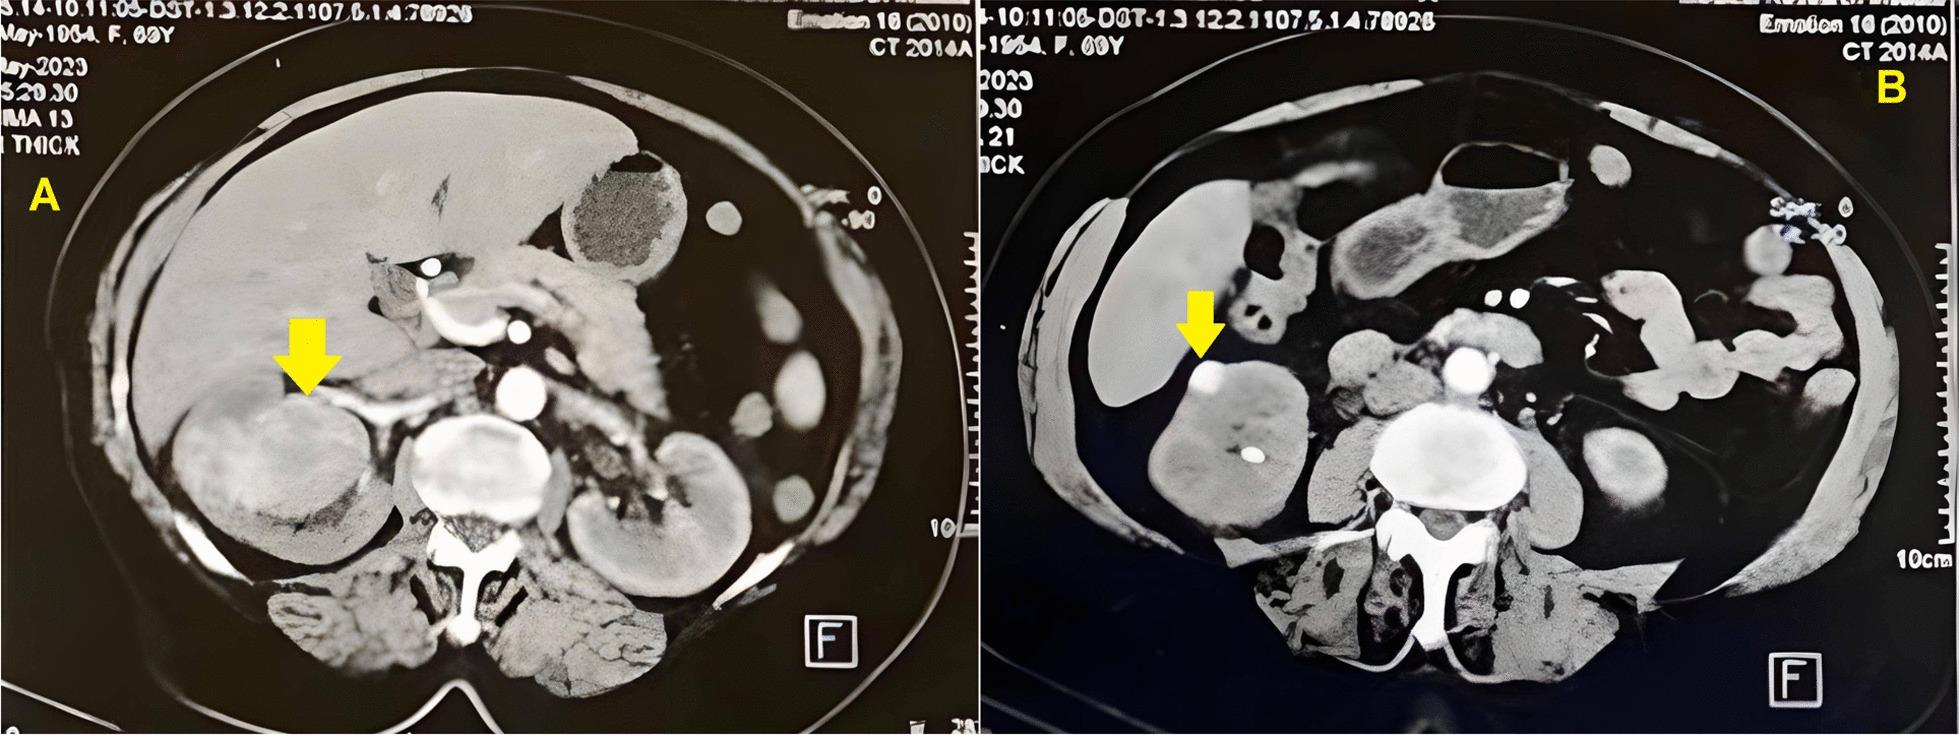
Multi-slice computed tomography displaying two right renal masses. A The first mass was an upper polar mainly anterior, totally endophytic, enhancing soft tissue mass lesion measuring 6.5 cm and invading the middle calyx. B The second smaller mass was a lower polar exophytic lesion measuring 1.3 cm
